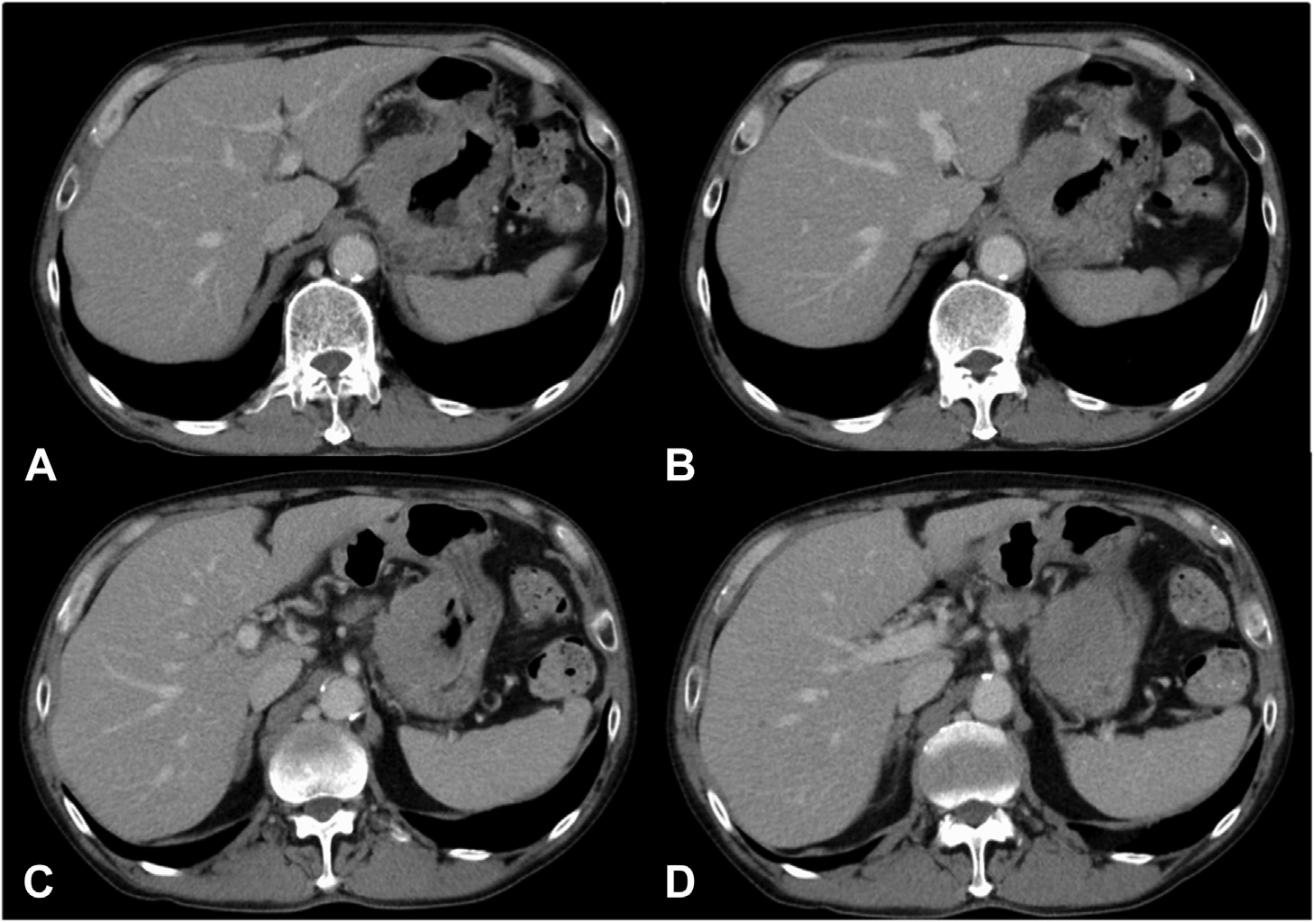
A plain CT scan of the abdomen showed an enlarged lymph node (C) on the lesser curvature side invading the stomach.
